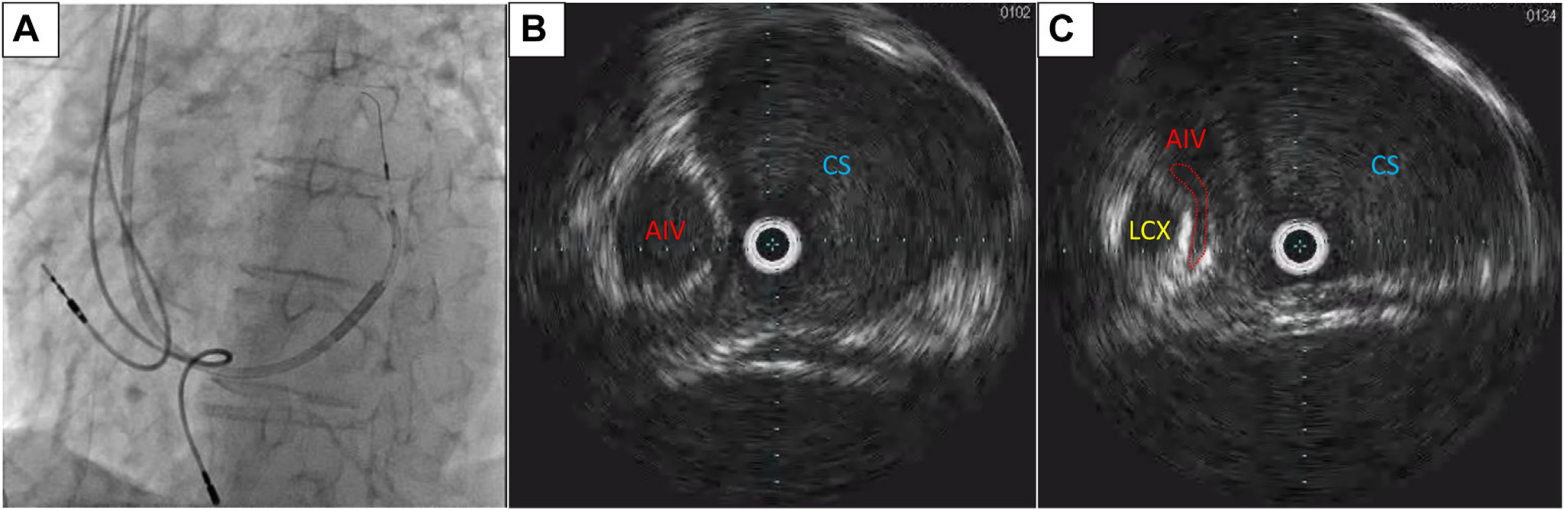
A: Fluoroscopy of intravascular ultrasound (IVUS) and guiding catheter at left anterior oblique view. B: IVUS image of the same level of (A). C: IVUS image close to anterior intraventricular vein (AIV). Note the AIV orifice is sandwiched between left circumflex (LCX) and coronary sinus (CS) trunk and shows eccentric narrowing.
